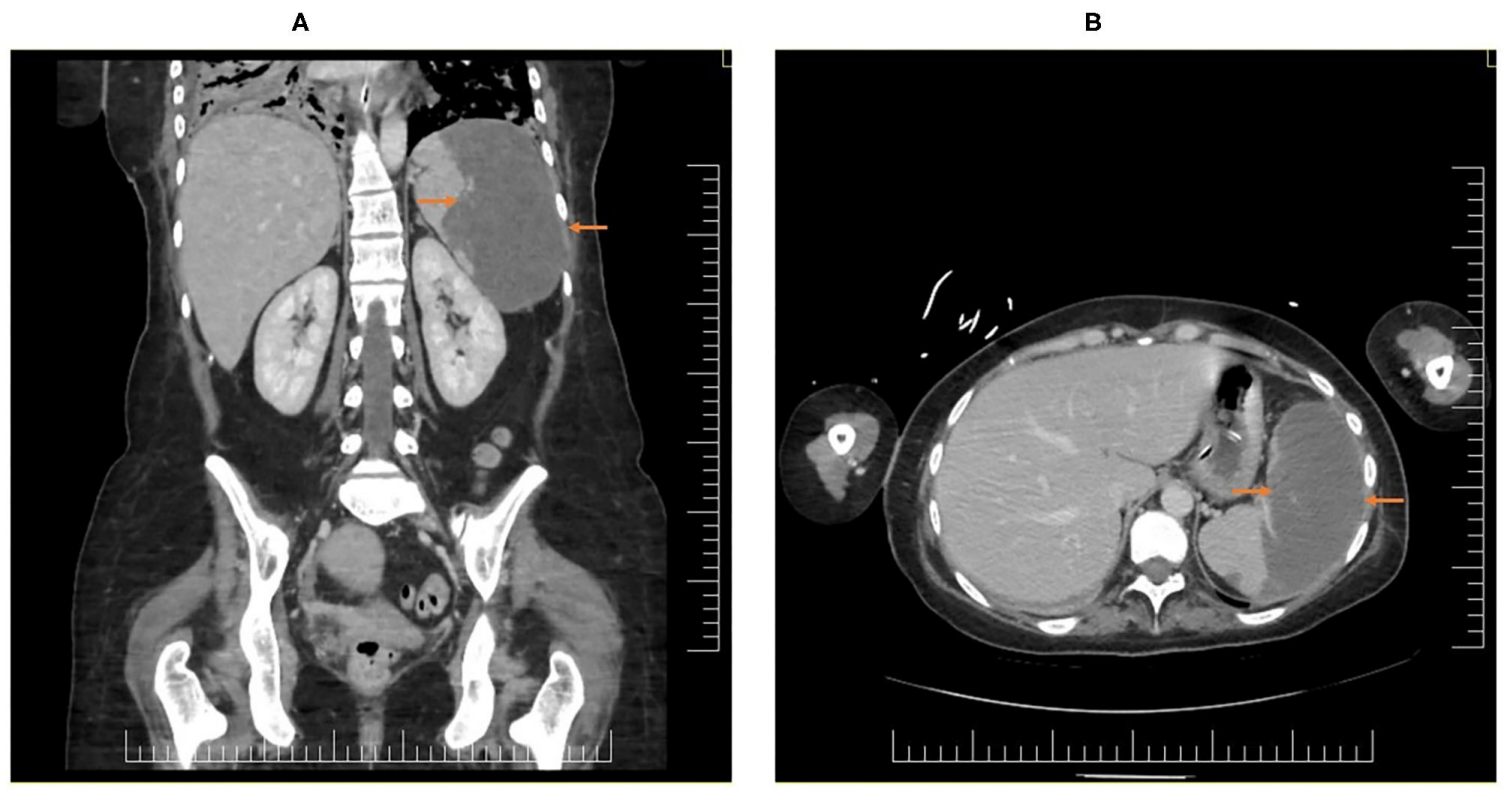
CT scan images showing the splenic infarction, (A) coronal plane and (B) axial plane.
